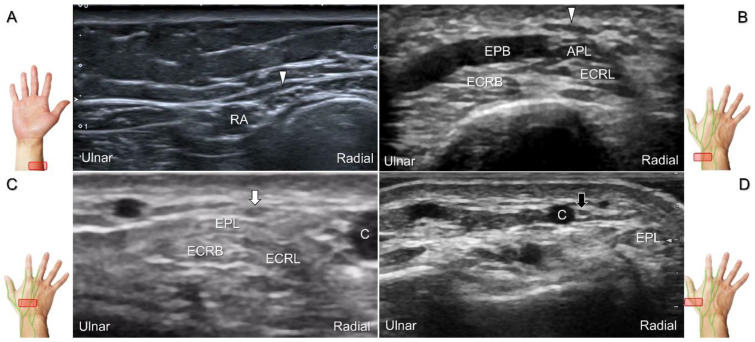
Sonographic imaging (short-axis view) of the superficial radial nerve from the distal third of the supinated forearm (A). With the pronated forearm, the nerve is seen to travel above the proximal intersection junction (B), divide into the dorsomedial branch coursing above the distal intersection junction (C), and the dorsolateral branch running beside the extensor pollicis longus tendon (D). Arrowheads: superficial radial nerve; white arrow: dorsomedial branch; black arrow: dorsolateral branch. RA: radial artery; APL: abductor pollicis longus tendon; EPB: extensor pollicis brevis tendon; ECRL: extensor carpi radialis longus tendon; ECRB: extensor carpi radialis brevis tendon; EPL: extensor pollicis longus tendon; C: cephalic vein.
